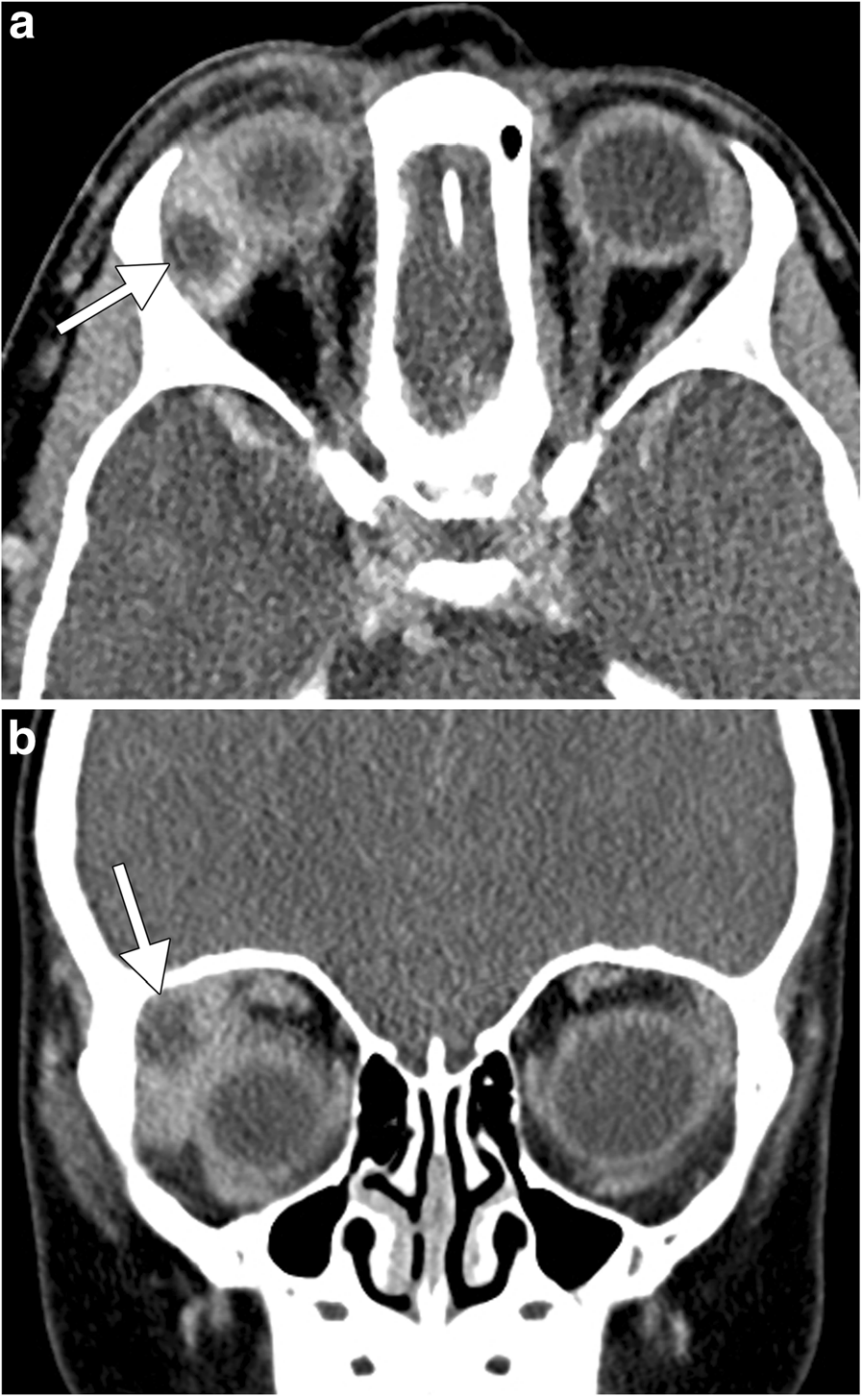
Axial (a) and coronal (b) contrast-enhanced CT images demonstrate right preseptal swelling and fat stranding as well as swelling and hyperenhancement of the right lacrimal gland, which contains a fluid collection (*arrow*)
